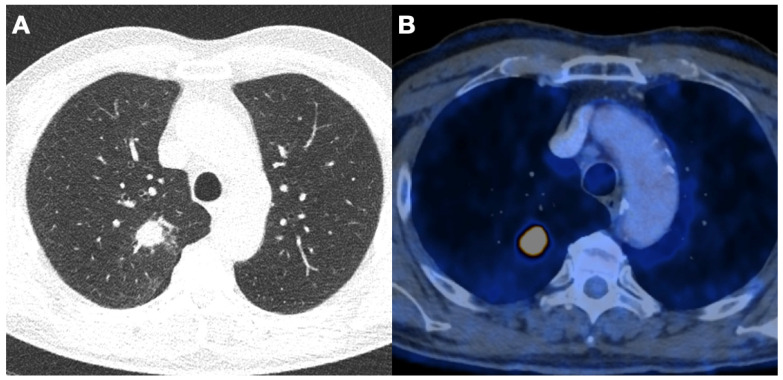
Low-dose CT (A) and FDG-PET/CT (B) images in axial plane of a 66-year-old male patient with stage IIIA squamous cell carcinoma in the upper right lobe. This previously asymptomatic patient underwent a lobectomy with systematic lymph node dissection and received adjuvant chemotherapy.
